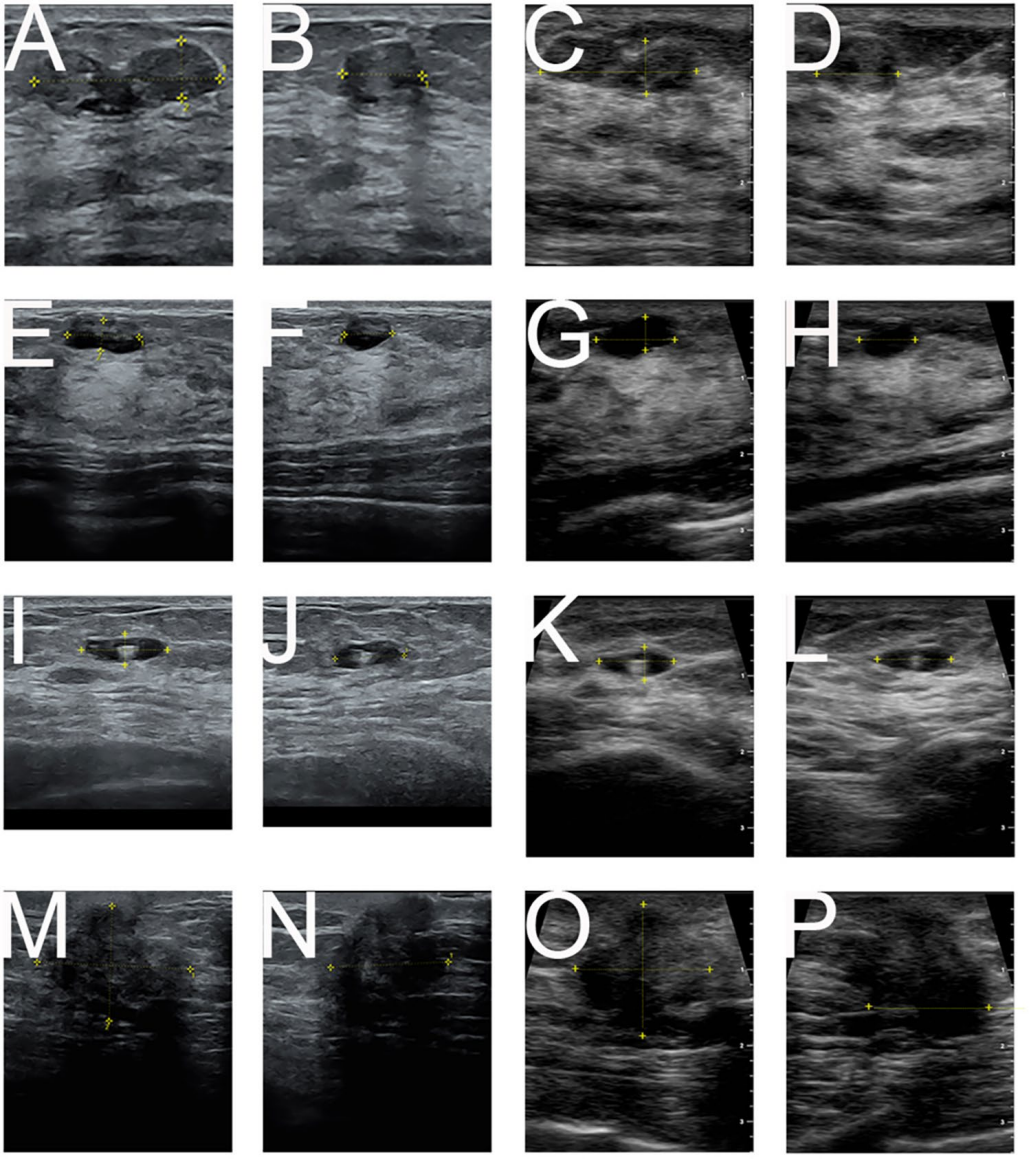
Ultrasound images of breast lesions: fibroadenoma (A–D), cyst (E–H), lymph node (I–L) and carcinoma (M–P) examined using a stationary high-end  ultrasound device (SHUD, A-B, E–F, I-J, M–N) and a handheld ultrasound device (HHUD, C-D, G-H, K-L, O-P)
